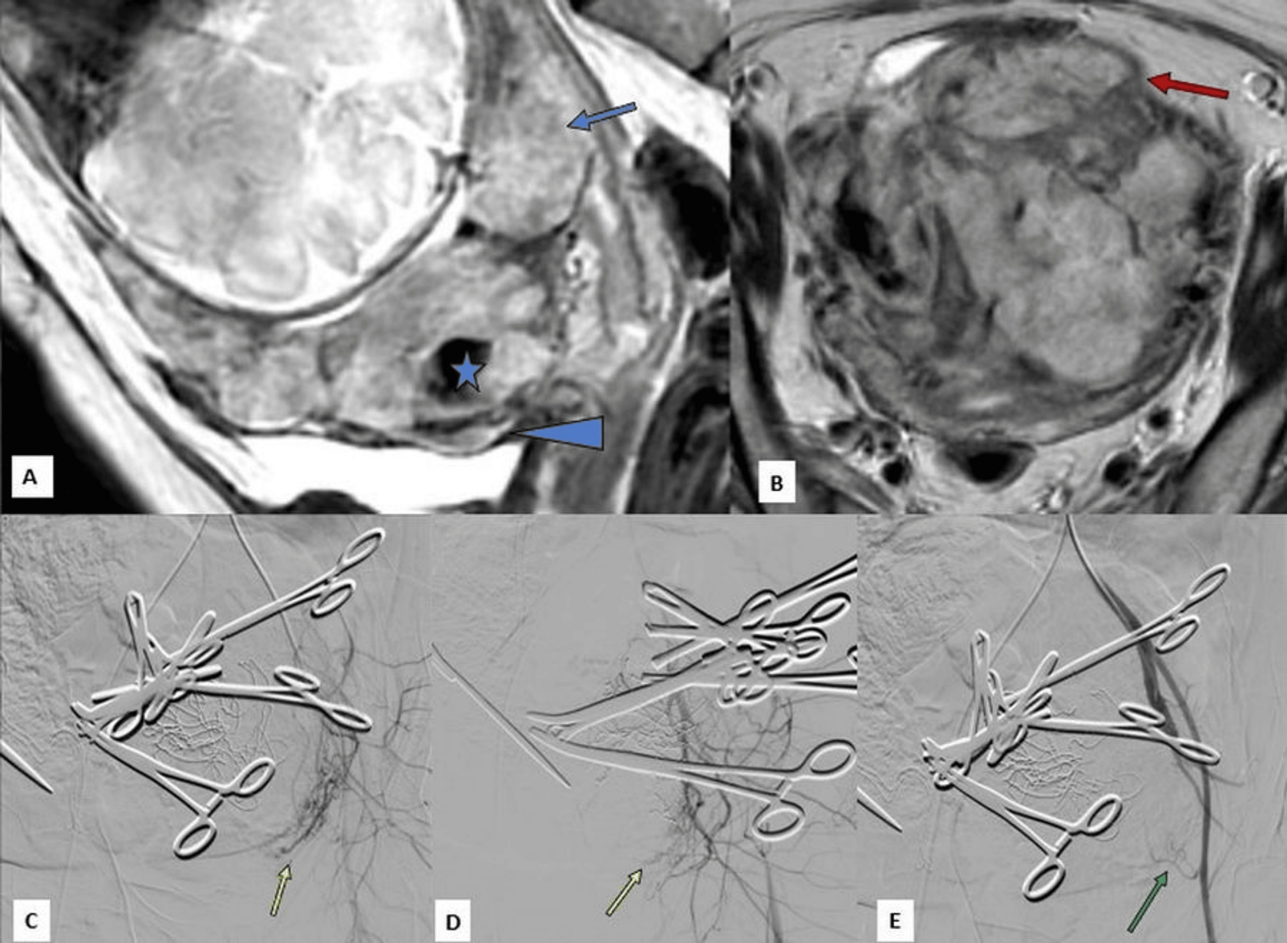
Magnetic resonance imaging of the pelvis with the placenta showing and internal iliac angiogram: A, sagittal section; B, coronal section; C-E, right internal iliac angiogram. (A, sagittal section) Grade 4 placenta previa (blue arrow) with intraplacental dark bands (blue star) and focal bulging of the anteroinferior uterine wall (blue arrowhead). (B, coronal section): The extension of the anteroinferior placenta up to the serosa and beyond (red arrow). (C-E) Internal iliac angiography, performed using a 5F Roberts uterine catheter (RUC), highlights inferior vesical arterial feeders (yellow arrow in C and D) supplying the placenta percreta. Post-embolisation angiography (E) confirms the complete obliteration of the abnormal vascular blush (green arrow).
